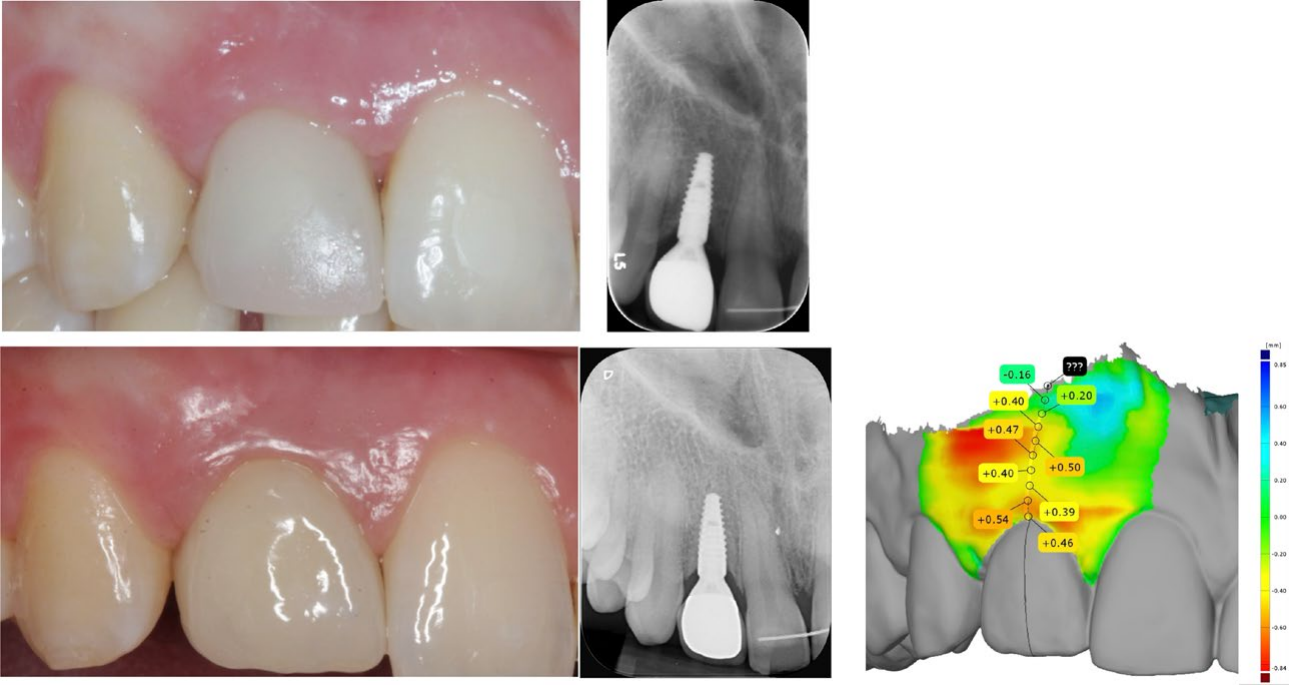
Clinical and radiographic presentation of a Ø3.3 mm implant at the 1‐ (a) and 5‐year (b) follow‐up along with the identified profilometric linear profile (c).
